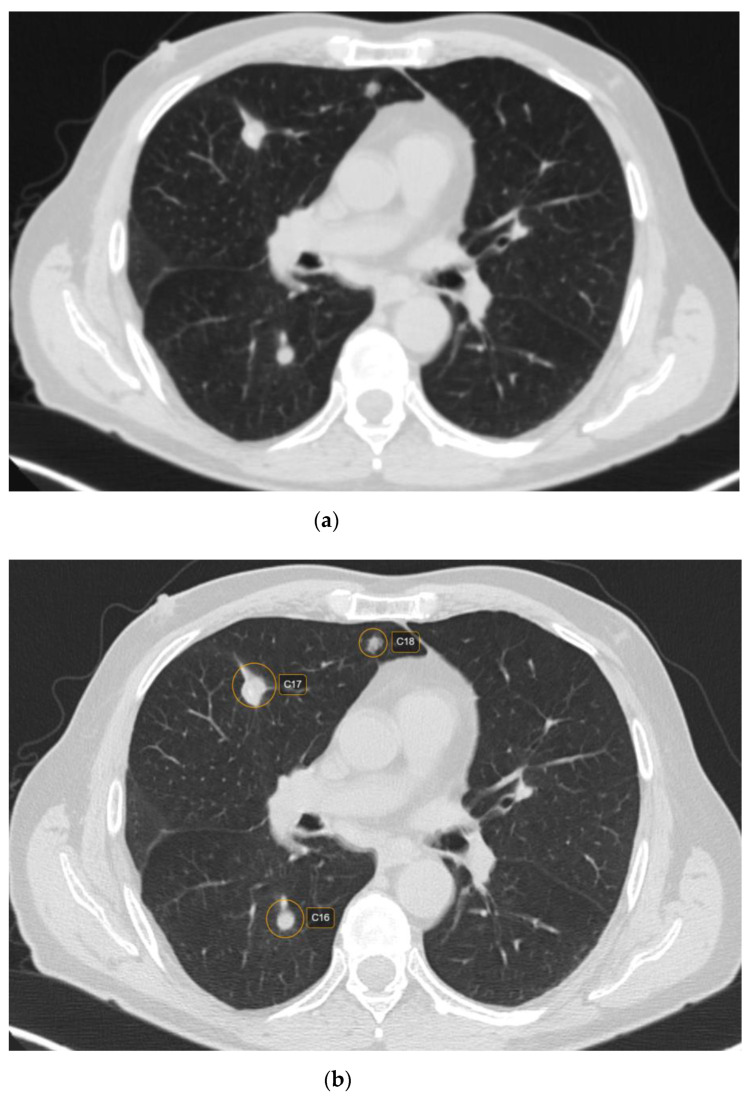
CT scan in lung window depicting lung nodules on raw CT images (a) versus marked CT images using CAD (b).
